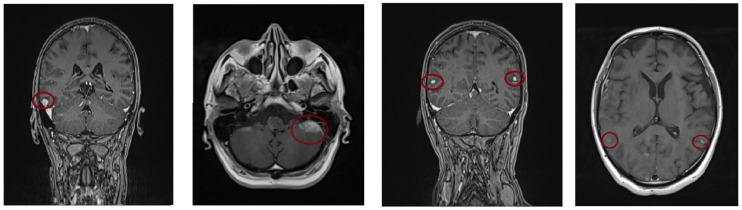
Brain MRI: multiple nodular meningeal lesions with intense contrast enhancement, located bilaterally in supratentorial and infratentorial regions, including the left cerebellar area. Imaging findings are suggestive of secondary meningeal involvement in the setting of multiple myeloma.
